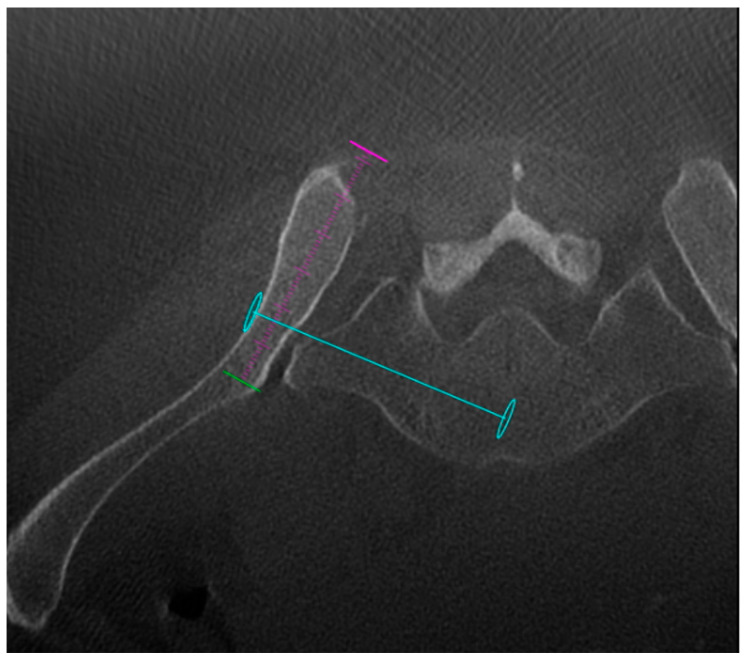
Initial CBCT images. Axial view shows the trajectory chosen by the operator, visible as a blue line, with the entry point at the iliac wing (the most lateral blue circle) and the distal point at the level of the S1 promontory (central blue circle). The purple and green circle indicates the projection line of the posterior iliac wing.
